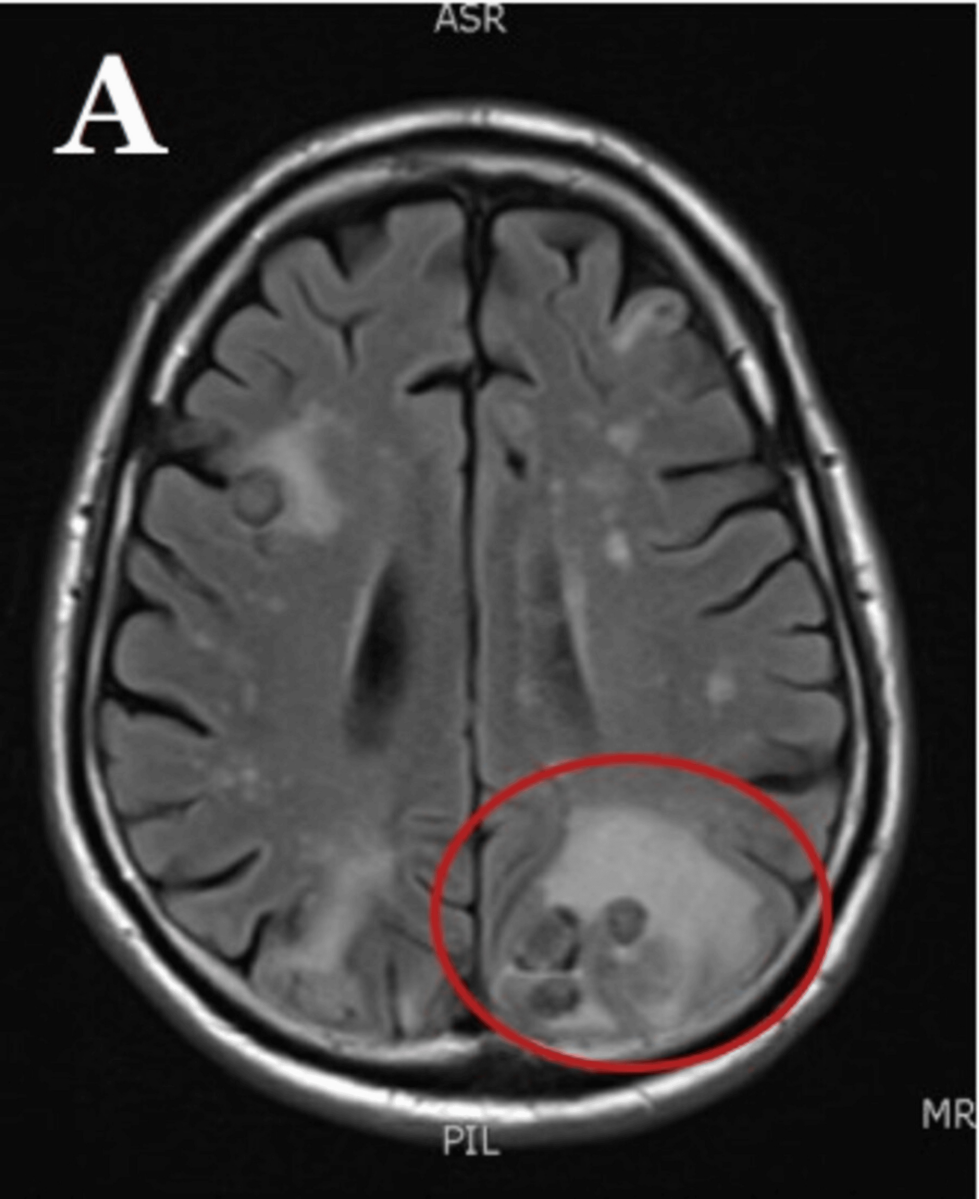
MRI brain demonstrating numerous enhancing brain lesions bilaterally, with the largest lesions including a 2.5 x 2.2 cm lesion in the left posterior temporal lobe
